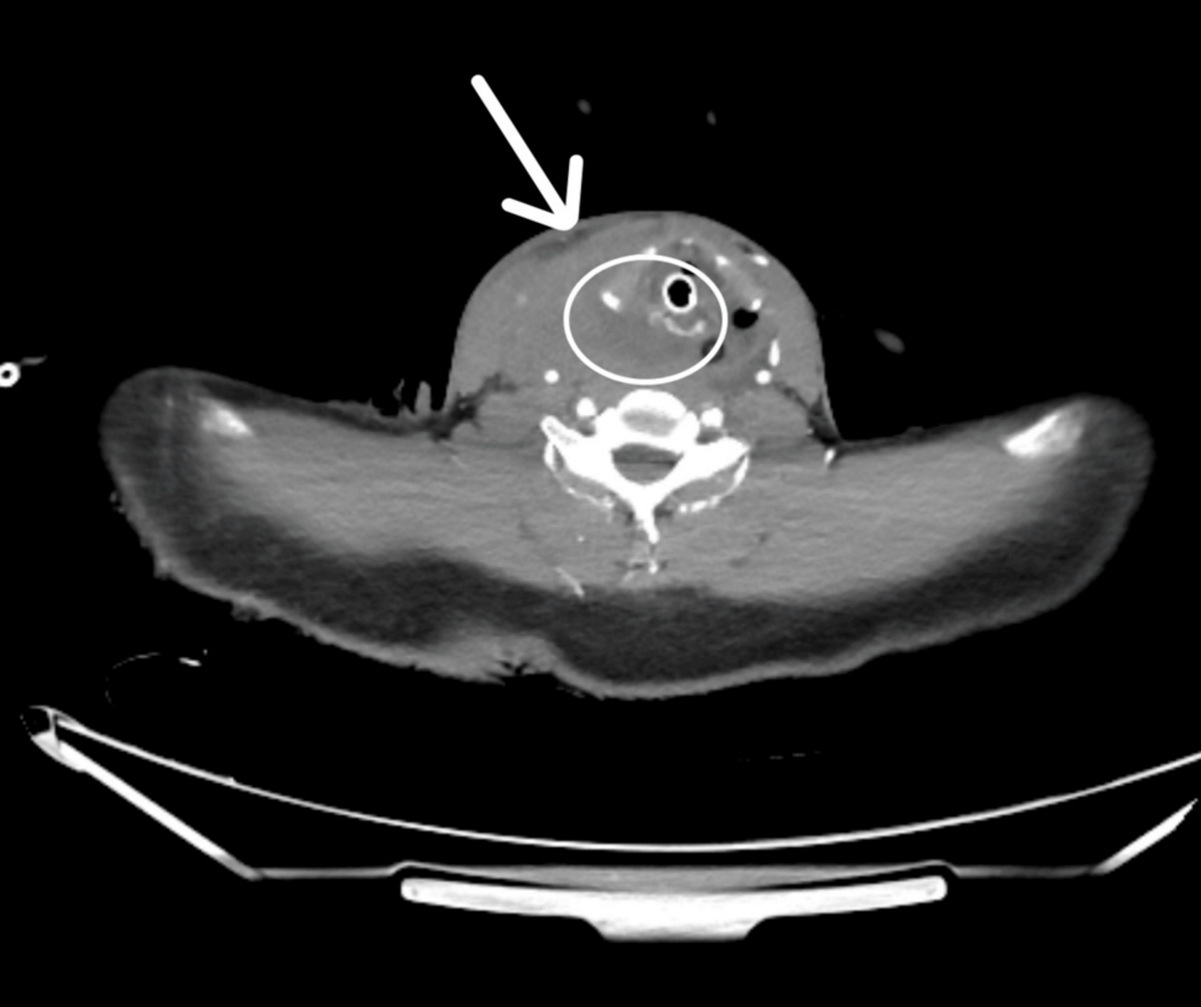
Contrast-enhanced CTA of the neck showing active extravasation from the right superior thyroid artery. CTA demonstrates contrast leak (arrow) from the right superior thyroid artery with surrounding cervical hematoma. CTA, computed tomography angiography
